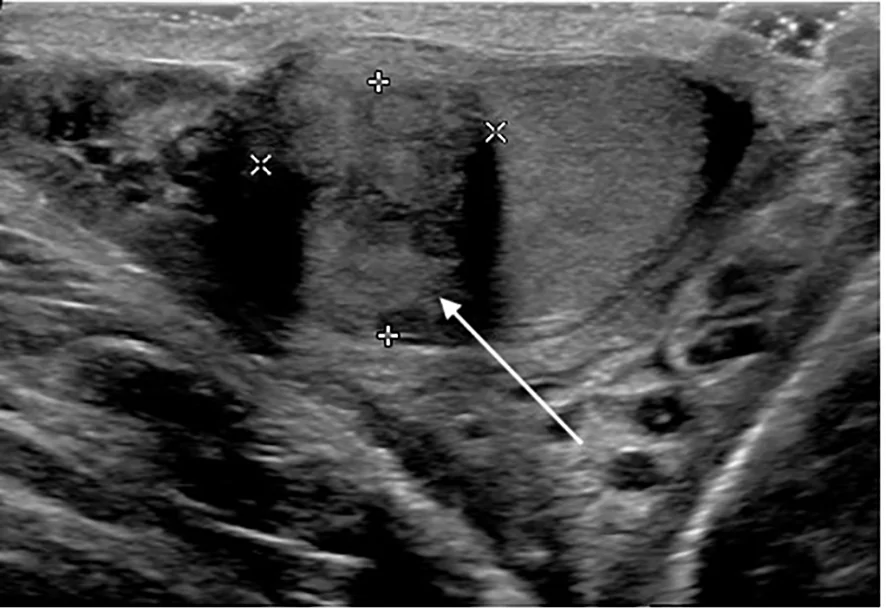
Gray-scale scrotal ultrasonography image, with the white arrow indicating an approximately 1.7 x 1.6 x 1.4 cm solid lesion in the superior pole of the right testis.
